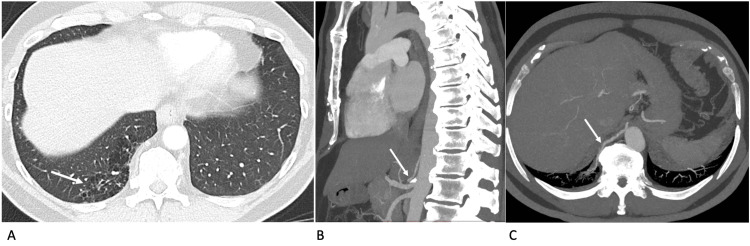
Case 4 - contrast-enhanced axial CT thorax Contrast-enhanced axial CT thorax in lung window (A), coronal mediastinal window maximum intensity projection (B) and axial mediastinal window maximum intensity projection (C). Pulmonary sequestration in the right lower lobe posteromedial segment is seen as an area of multiple cystic segments (arrow in A) supplied by an arterial branch arising directly from the celiac trunk (arrow in B). Note the course of the supplying artery coursing to the sequestration (arrow in C).
